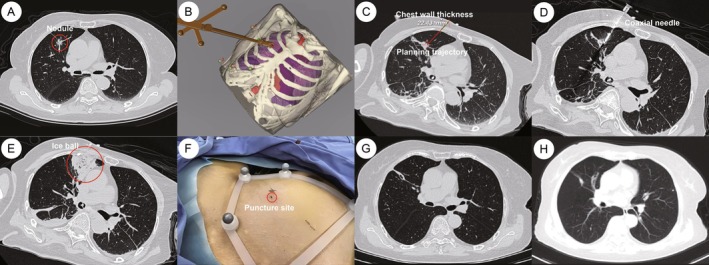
(A–H) Follow‐up and robotic‐assisted CRA procedural images of a 77‐year‐old woman diagnosed with a pulmonary sub‐solid nodule. (A) Initial CT scan image, showing a 14‐mm‐sized mixed GGN involving the anterior segment and apical segment of the right upper lobe (red circle). (B) 3D model reconstruction of the thoracic structure. (C) Planning of the needle trajectory (red line) based on the initial CT scan. (D) Intra‐procedure imaging, showing coaxial needle inside the lesion during ablation procedure. (E) Post‐procedure imaging, showing an ice‐ball of sufficient size (red circle) completely ablates the nodule. (F) Minimal trauma with only a small entry point (red circle) on the skin of our patient (black mark indicated a comparison with conventional free‐hand manipulation). (G) Three‐month follow‐up imaging, showing a scar formation in the ablation zone. (H) Seven‐month follow‐up imaging, showing a successful ablation with no recurrence. 3D = three‐dimensional, CRA = cryoablation.
